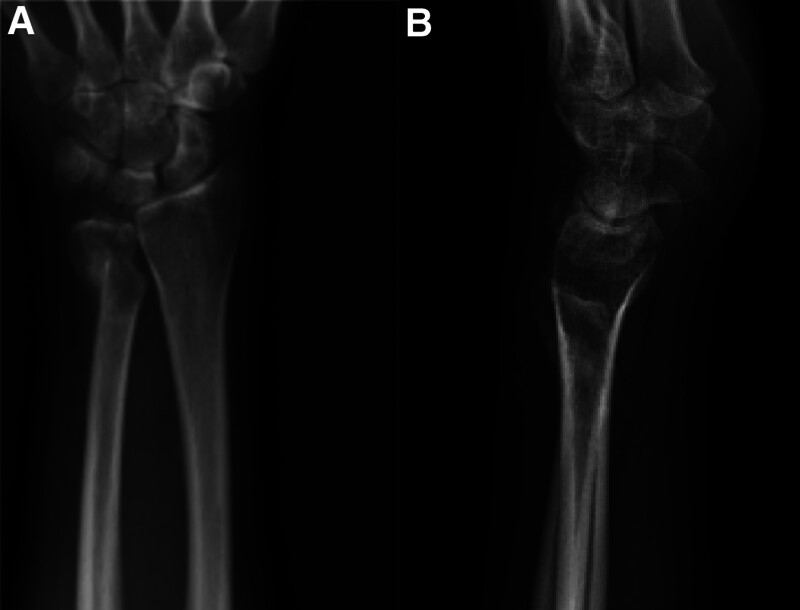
X-ray demonstrated a pathological fracture in the distal ulnar. (A) Anteroposterior view; (B) lateral views.
